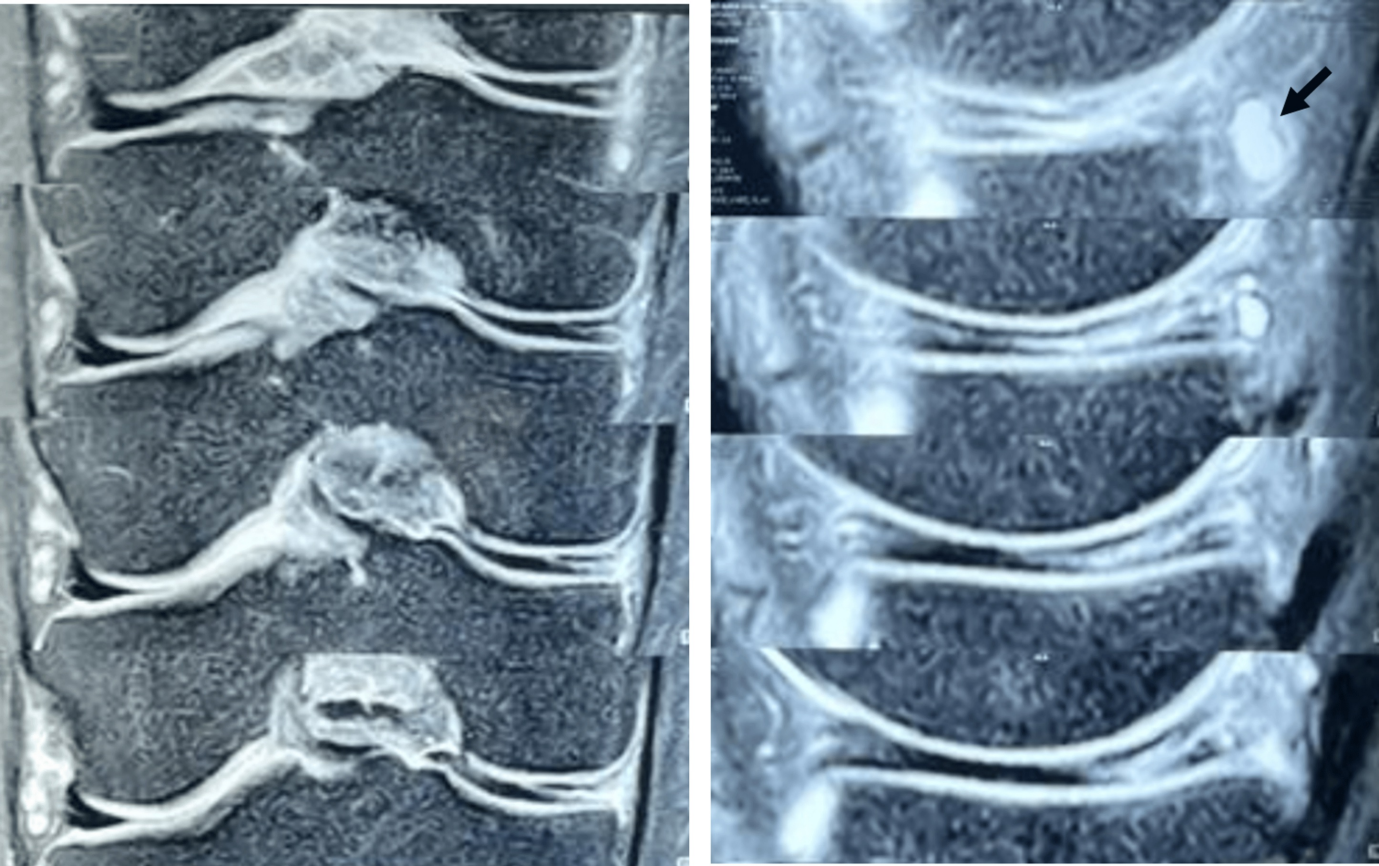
T2-weighted MRI showing the medial discoid meniscus in coronal (left) and sagittal (right) views, with the characteristic "bow-tie" sign evident in the sagittal view. The meniscal cyst is highlighted (arrow).
